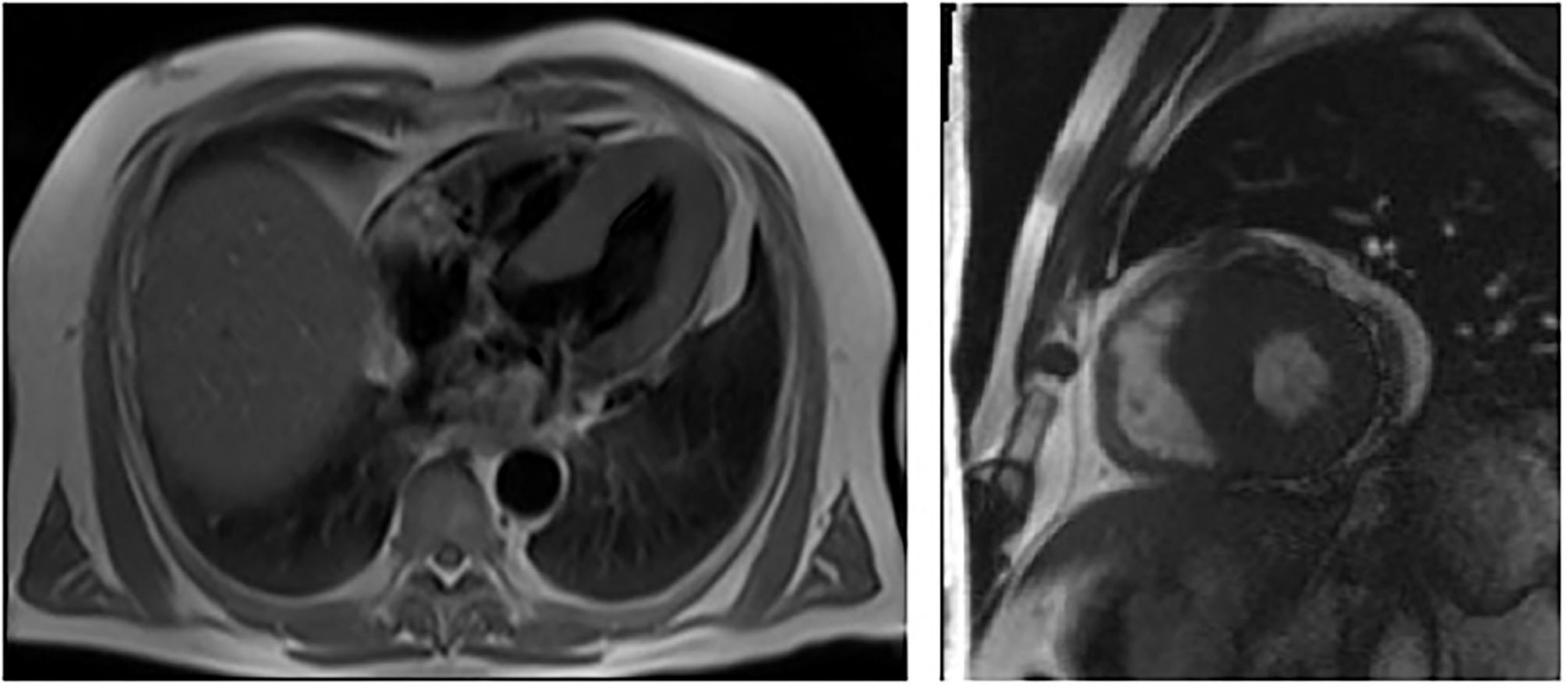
Cardiac MRI showing left ventricular hypertrophy with no late enhancement of Gadolinium in patient 1 (III – 13, proband).
